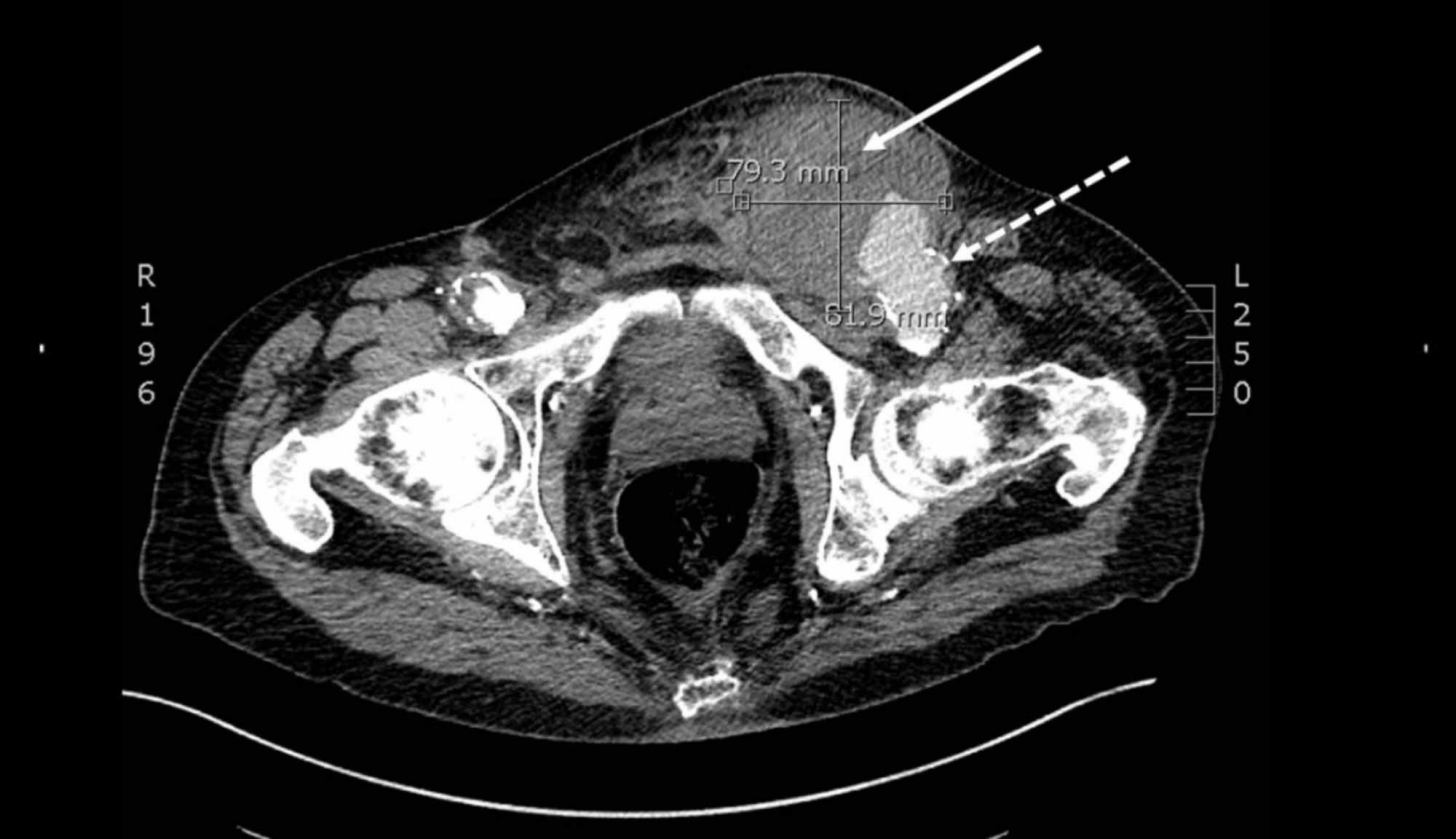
A transverse slice of a computed tomography pelvis angiogram demonstrating an 8.2 x 7.9 cm left common femoral artery (dashed white arrow) pseudoaneurysm (solid white arrow) with active extravasation
